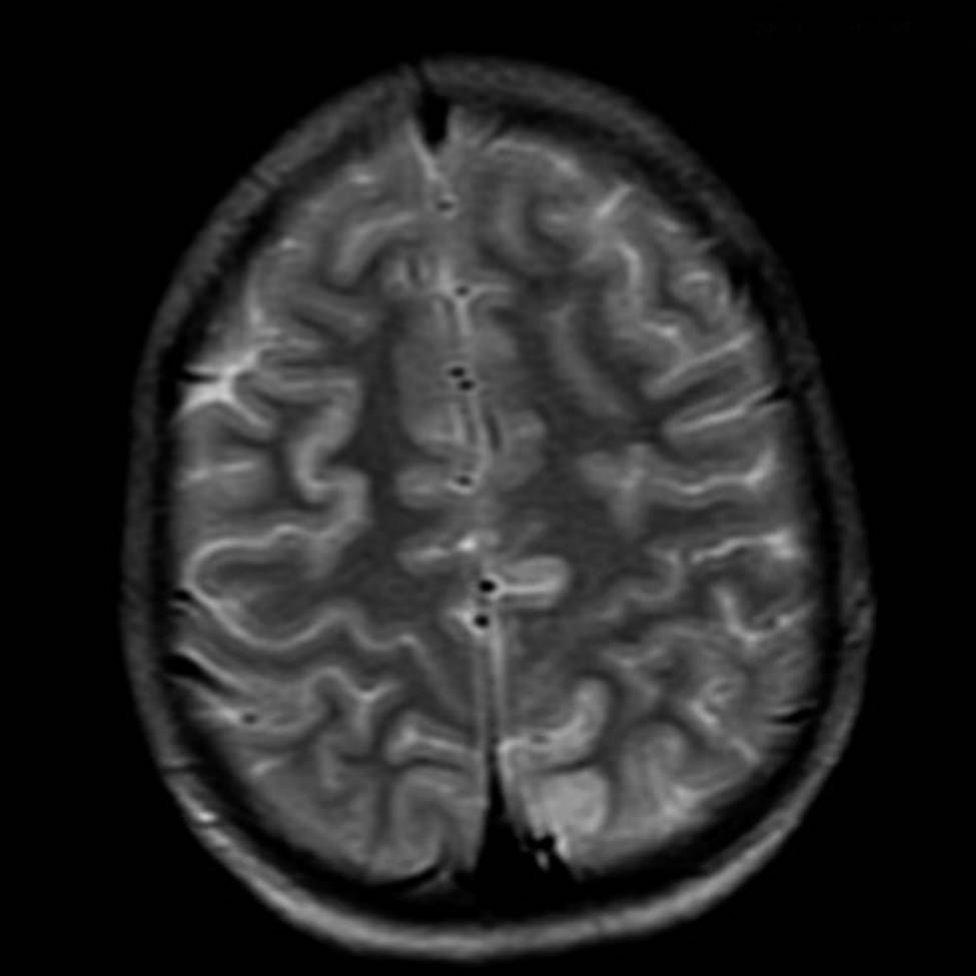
Axial view of MRI Brain showing subtle T2 hyperintensities in left frontal and bilateral parietal cortical sulci and subcortical white matter.
